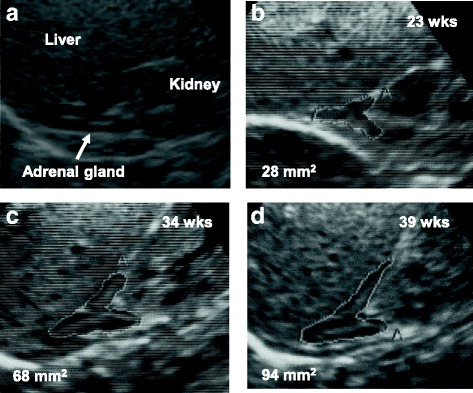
(a) Ultrasonographic scan of the right adrenal region of a representative neonate obtained immediately after birth by the longitudinal antero-lateral approach. Sonographic appearances of the right adrenal gland of a representative in each group: (b) group 1: a neonate born at 23 weeks of gestation, (c) group 2: a neonate born at 34 weeks of gestation, and (d) a neonate born at 39 weeks of gestation. The border of the adrenal gland was traced manually to calculate the adrenal gland area
